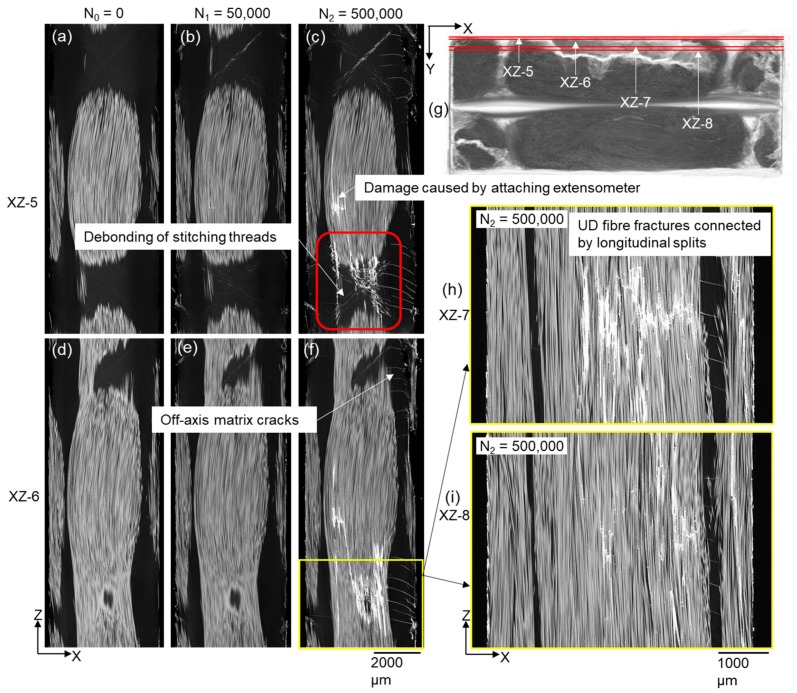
(a–f) and (h,i) Sub-surface X-ray CT virtual XZ sections showing the development of UD fibre fractures from the debonding of stitching threads. The Y-positions of the XZ slices are shown in (g).
